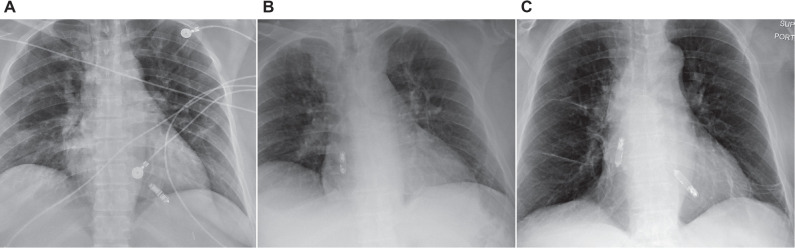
Frontal chest radiograph of leadless permanent pacemaker (PPM) options. A: A single-chamber leadless PPM in the right ventricle. B: A single-chamber leadless PPM in the right atrium. C: A dual-chamber PPM in the right atrium and ventricle.
